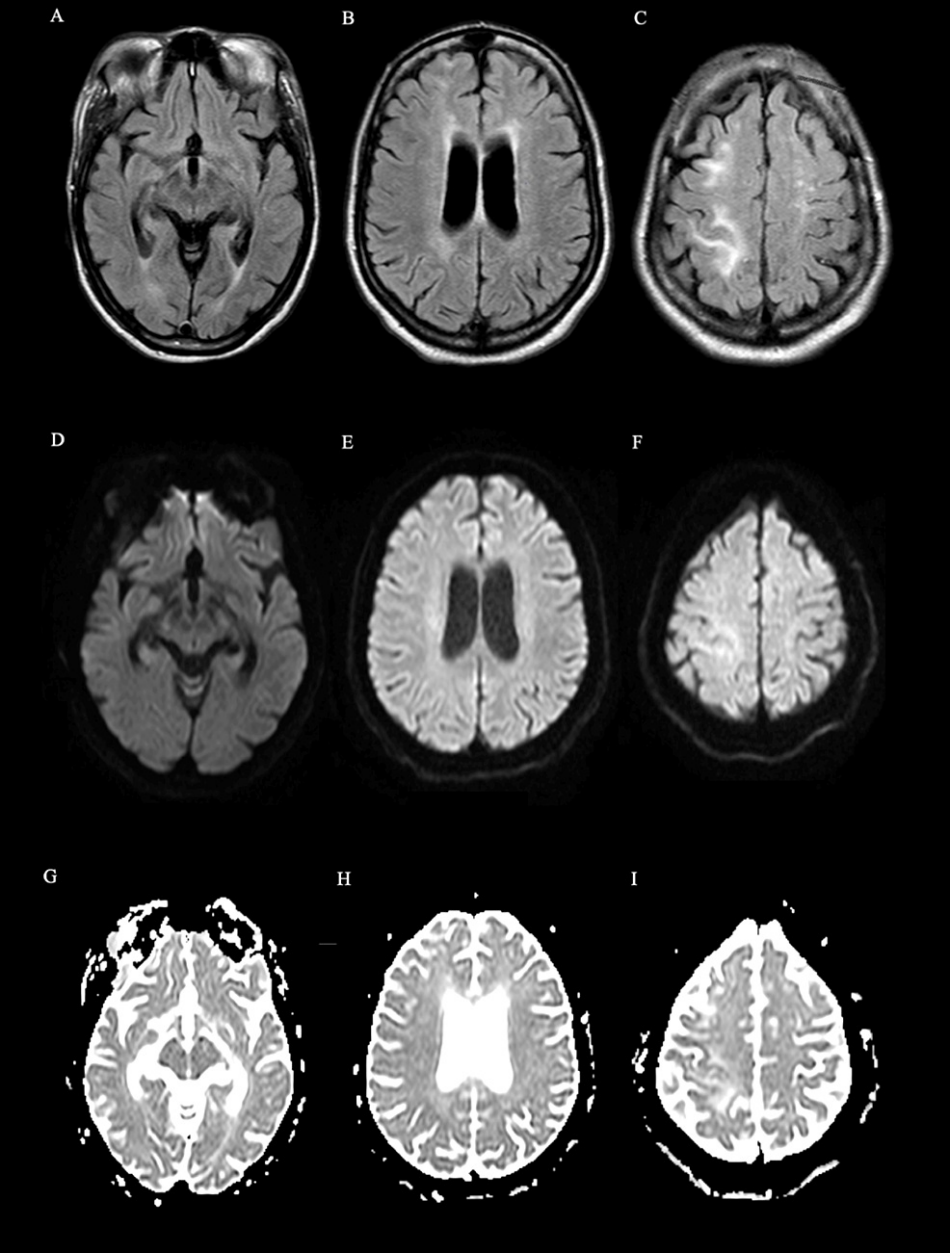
(A-I) Axial MR images performed on day nine demonstrated significant interval improvement of the vasogenic edema. (A-C) FLAIR sequence showing the interval improvement of hyperintense lesions seen on previous imaging. (D-F) DWI sequence showing interval resolution of previously seen focus of restricted diffusion. (G-I) ADC sequence showing interval improvement of hyperintense lesions seen on previous imaging. Magnetic resonance (MR); fluid-attenuated inversion recovery (FLAIR); apparent diffusion coefficient (ADC); diffusion-weighted imaging (DWI)
